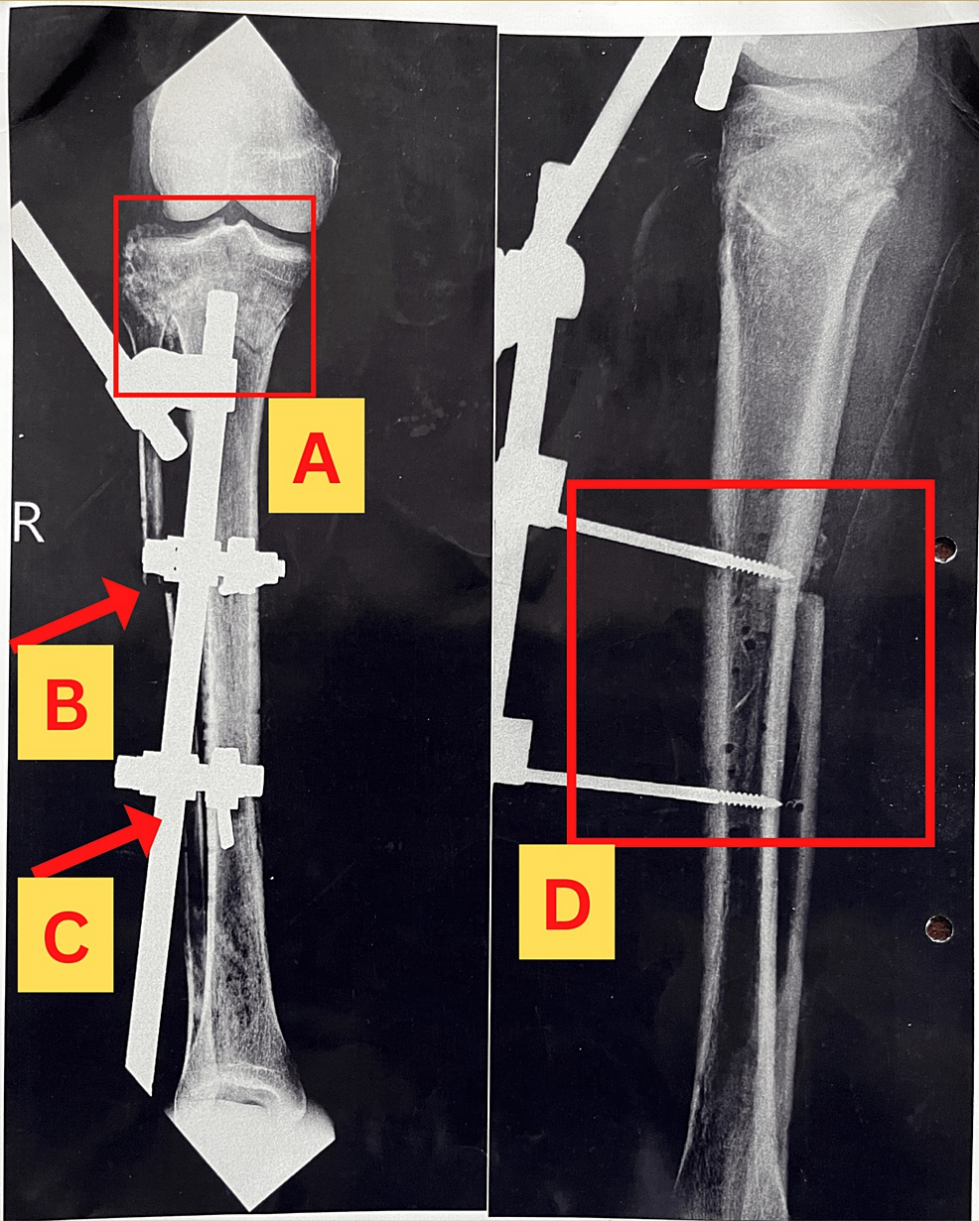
X-ray showing the anteroposterior view (left) and lateral view (right) of the right leg obtained after admission to the orthopedic ward. The square marked as "A" shows the healing proximal tibial fracture associated with the fibular fracture pointed by the arrow marked as "B." Non-bridging external fixator is pointed by arrow "C." The left side of the image represents a radiograph of the anteroposterior view of the leg in which the square marked as "A" shows the healing proximal tibial fracture associated with the fibular fracture pointed out by the arrow "B", whereas the non-bridging external fixator is pointed by the arrow. The right side of the image represents the lateral view of the leg showing external fixation sites along with bony erosions of the tibia sowed in the square marked as "D."
